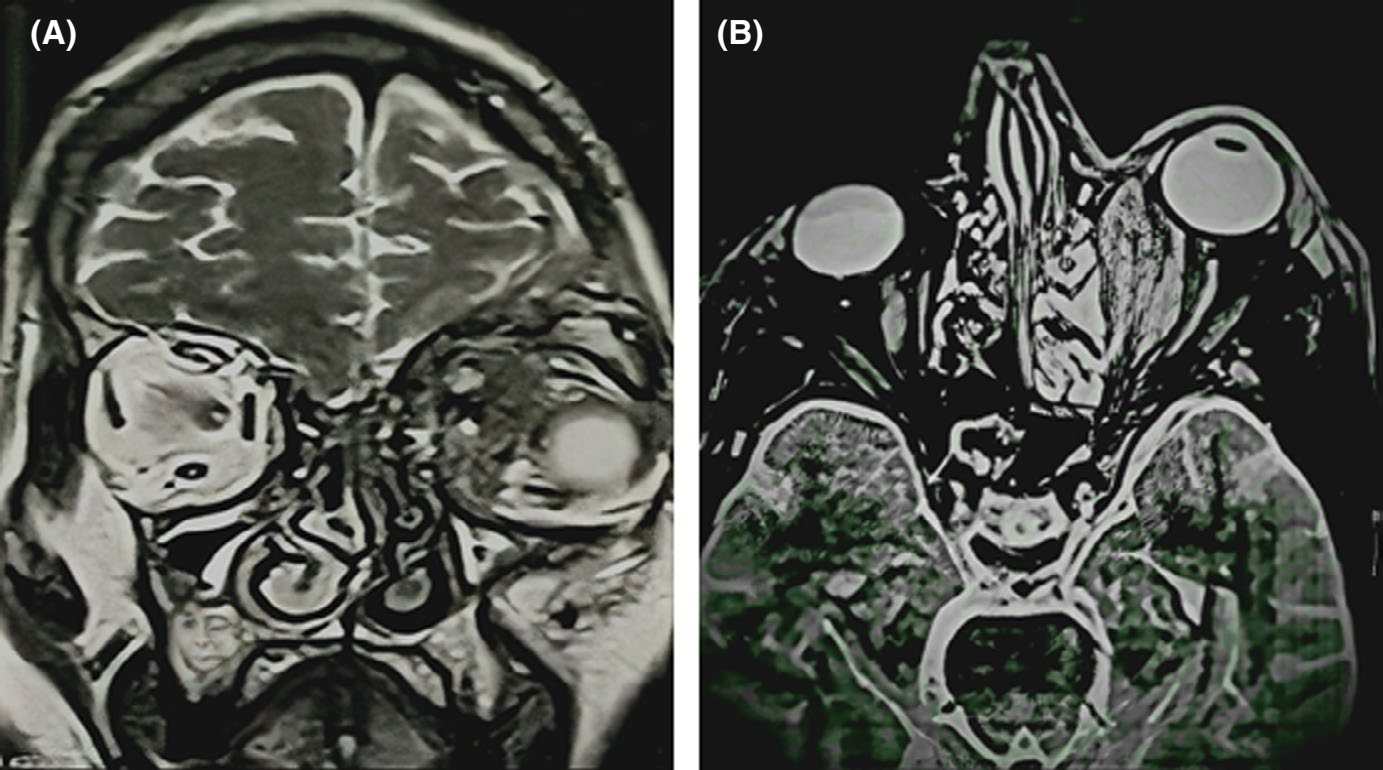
Brain magnetic resonance imaging (MRI) coronal T2‐WI (A) and axial T2‐WI (B) showing the large orbital mass surrounding and indenting the left globe
